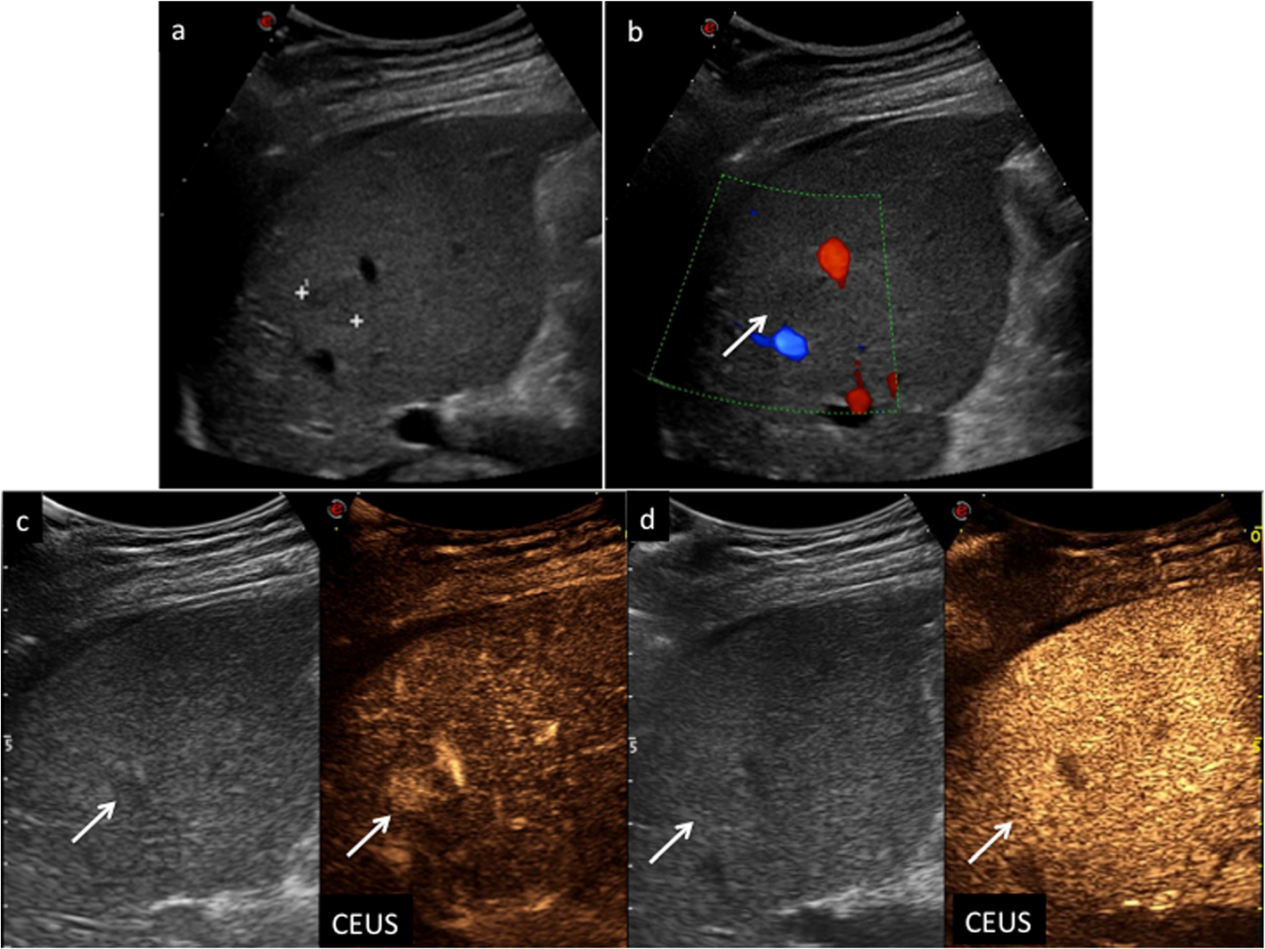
CEUS LI-RADS 4 (probably HCC). Nodule in a 52-year-old woman with virus C-related cirrhosis. Baseline US image (a) shows a slightly hypoechoic lesion sized 1.5 cm in the segment VIII (calipers). No vascular signal is detectable at color-Doppler (b) (arrow). At CEUS, the nodule is highly hypervascular during the arterial phase (c) (arrow) and isoechoic in the extended portal venous phase (d) (arrow)
